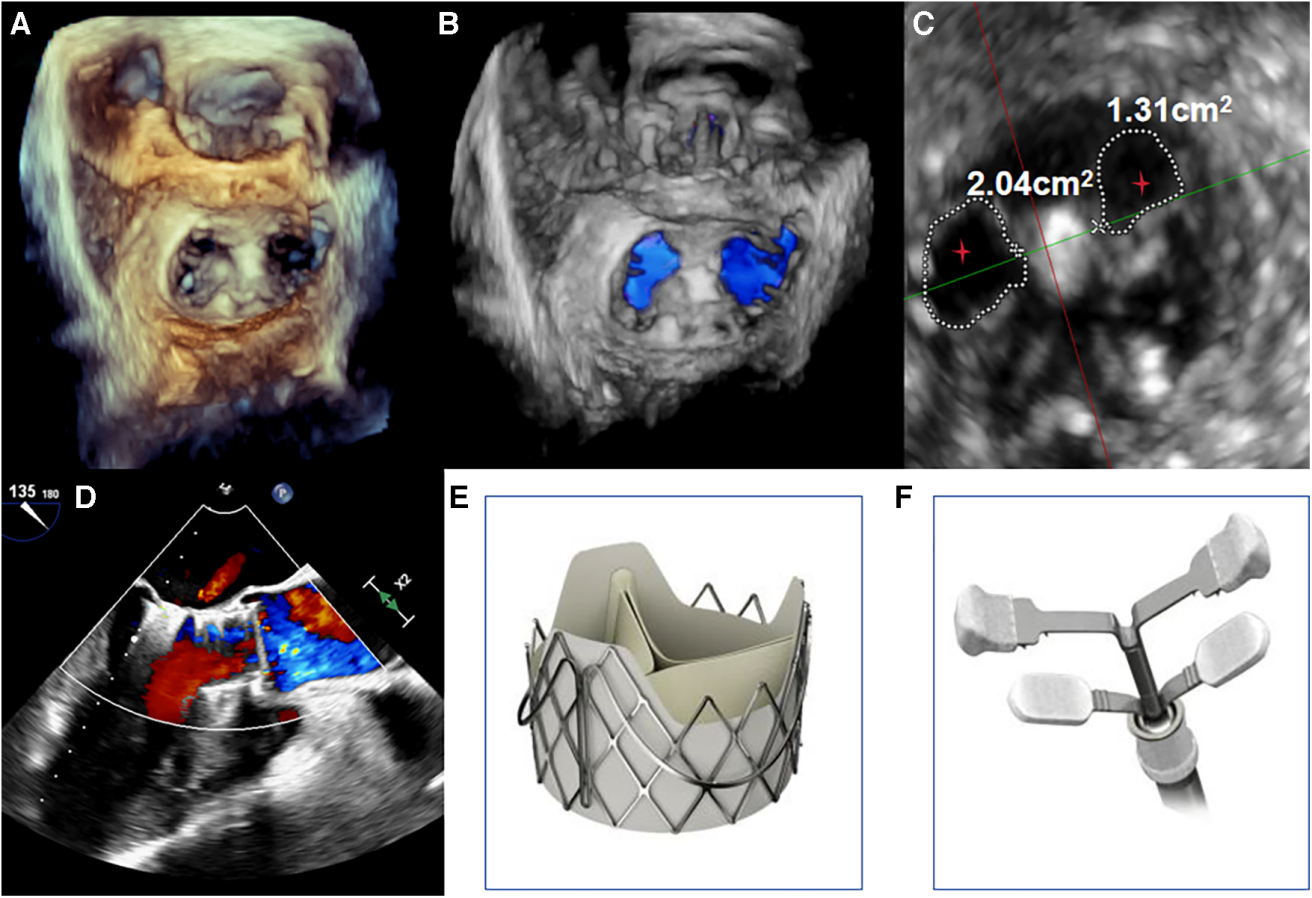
(A,B) under transoesophageal echocardiography guidance, one clip was implanted between the A2 and P2 leaflets. Transoesophageal echocardiography with 3D reconstruction demonstrated appropriate morphologies of the mitral and aortic valves post-TAVR and transcatheter edge-to-edge repair. (C) The mitral valve dual orifice areas measured by transoesophageal echocardiography were 2.04 cm^2^ and 1.31 cm^2^, respectively. The red stars in Figure depict the dual orifice areas of the mitral valve. (D) Transoesophageal echocardiography showed mild paravalvular regurgitation of the aortic valve after TAVI and TEER, with resolution of MR. The maximum transvalvular pressure gradient across the mitral valve was 3 mmHg, with a mean pressure gradient of 1 mmHg. (E,F) The transcatheter heart valves used were the J-Valve (JieCheng Medical, China) for the aortic position and the MVC-IIIf mitral valve clip (Shanghai Hanyu Medical, China) for the edge-to-edge repair.
